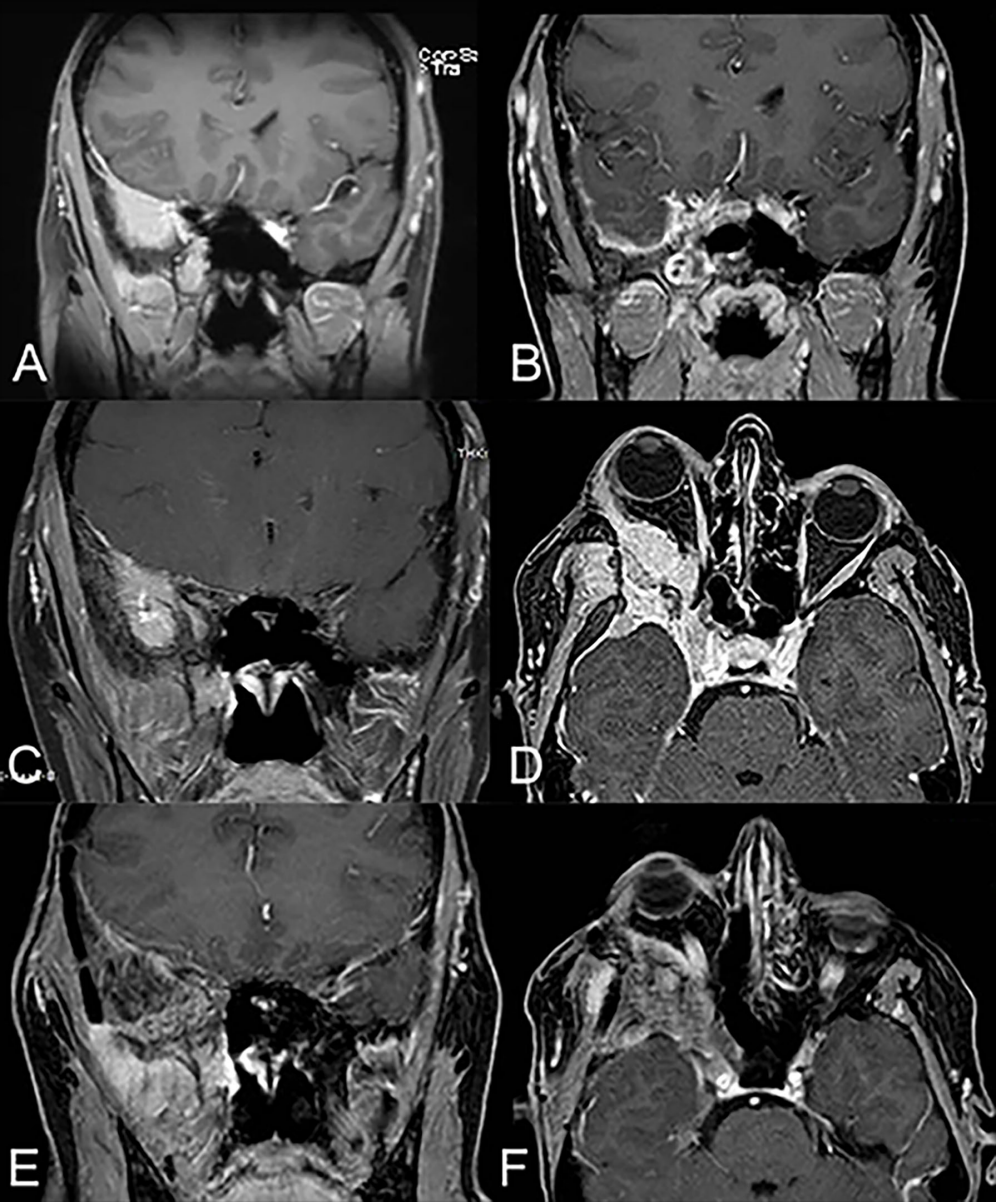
A 48-year-old woman affected by right spheno-temporal meningioma (pre-operative MRI depicted in (A)) was submitted to endoscopic-assisted resection *via* combined trans-nasal and trans-orbital corridors, obtaining gross total resection (post-operative MRI depicted in (B)). During the follow-up, 5 years after the primary treatment, she developed right proptosis and periorbital pain and the MRI documented a recurrence of the meningioma (MRI in panel (C) involving also the transorbital corridor (MRI in panel (D). The patient was then submitted to revision surgery *via* transcranial approach (right frontotemporal orbitozygomatic craniotomy). The MRI performed two years after revision surgery were clear from macroscopic recurrence of disease (E, F), with partial resolution of the right orbital pain and proptosis of the patient.
